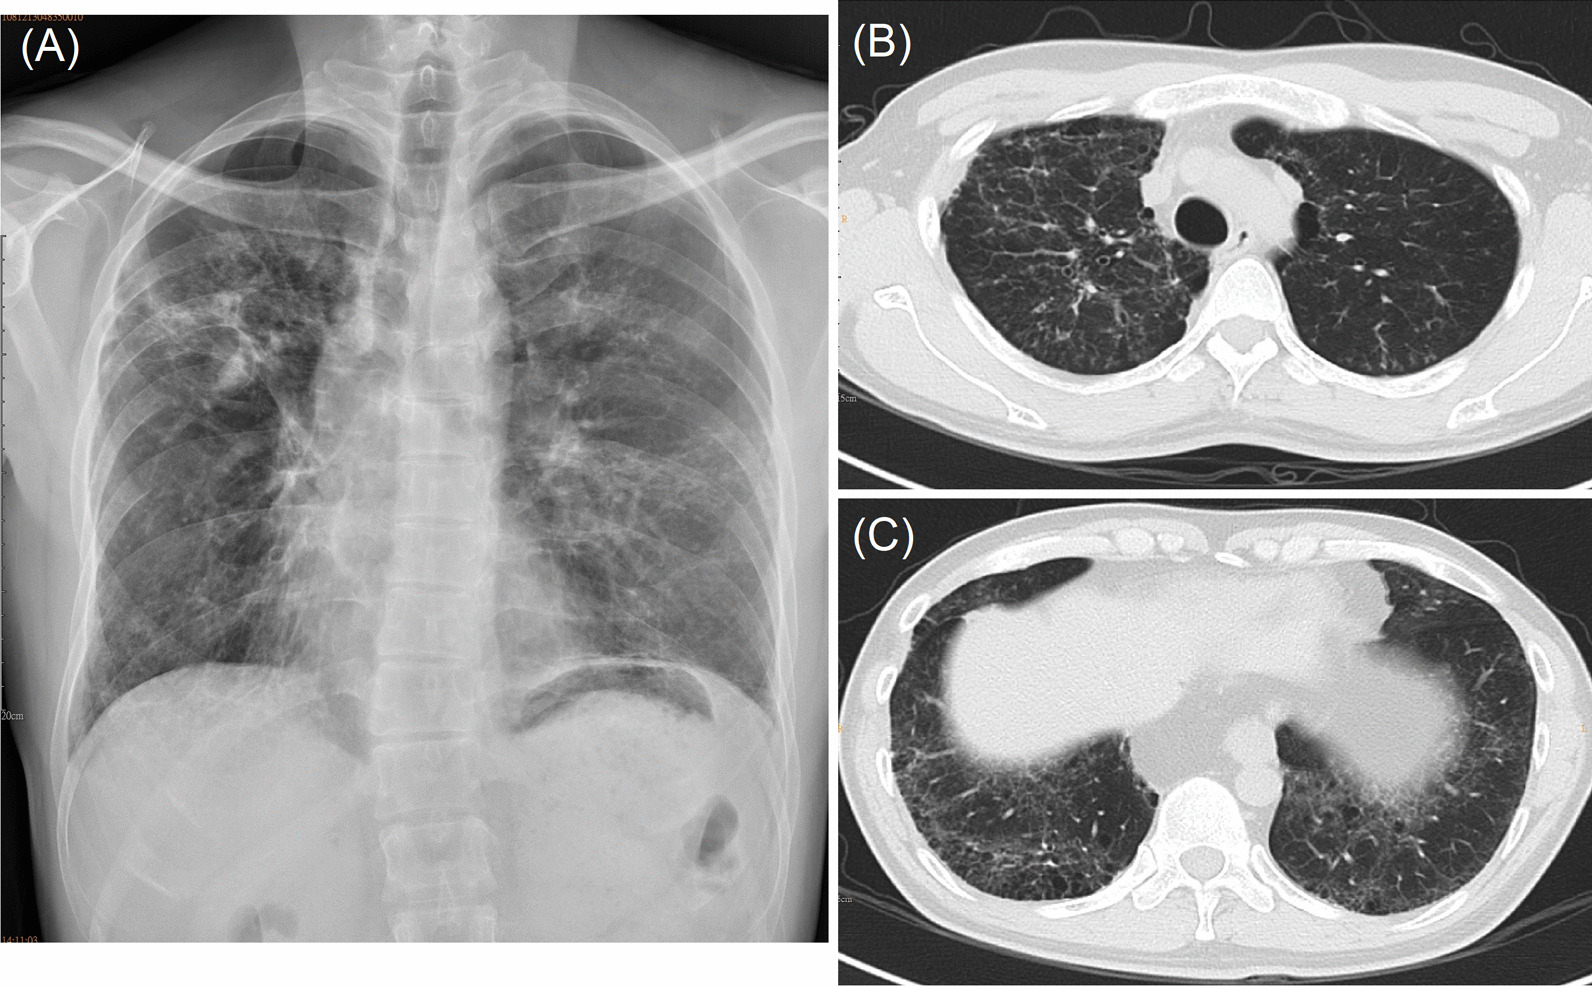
A The chest radiograph and B high-resolution computed tomography images (upper and lower lungs) of the first patient revealed nodular infiltration in the bilateral upper lungs, and C diffused honeycombing in the bilateral lung bases
